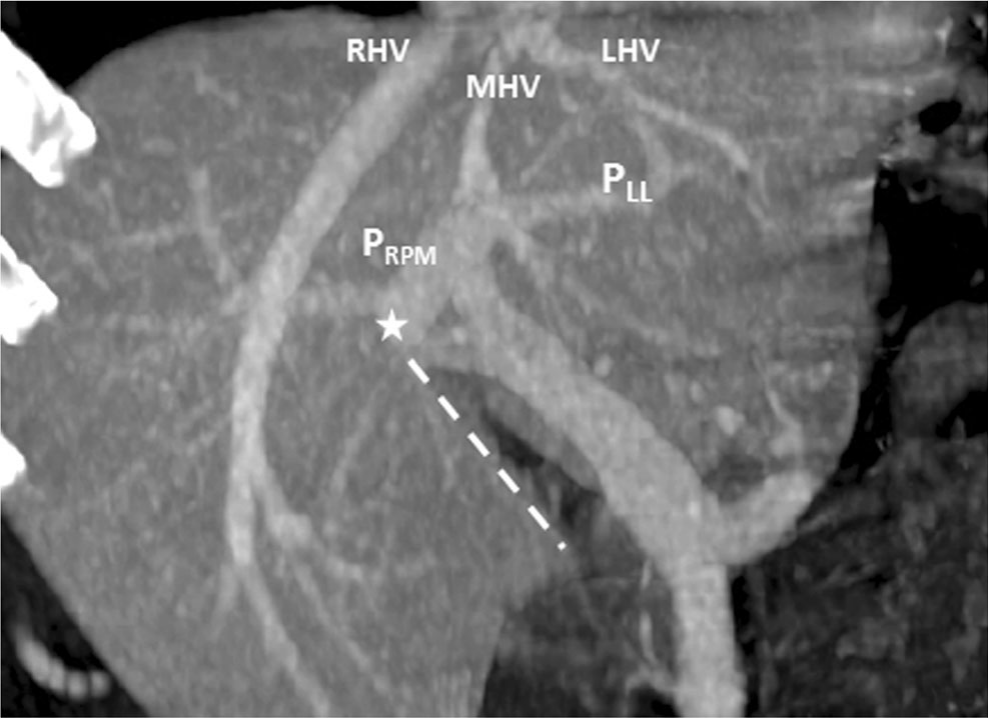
Contrast-enhanced CT scan revealing the right paramedian portal pedicle (P_RPM_) forming the right umbilical portion of the portal vein (*star*) and joining the right-sided ligamentum teres (RSLT) (*dotted line*), with the middle hepatic vein (MHV) running to the left of the umbilical portion and RSLT
